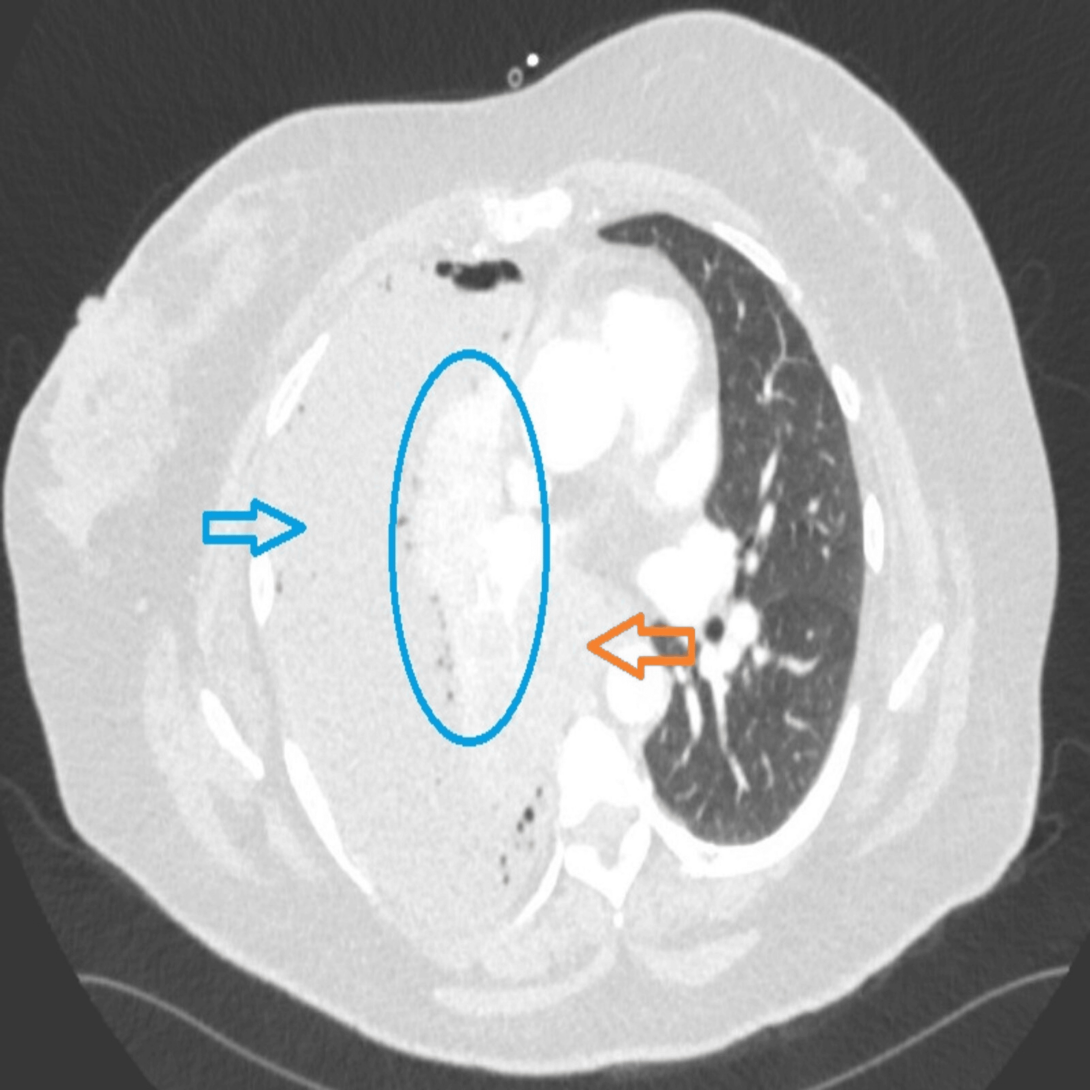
CT chest shows large empyema. The blue arrow indicates empyema, the collapsed lung is shown by the blue circle, and paratracheal invasion is indicated by the orange arrow.
